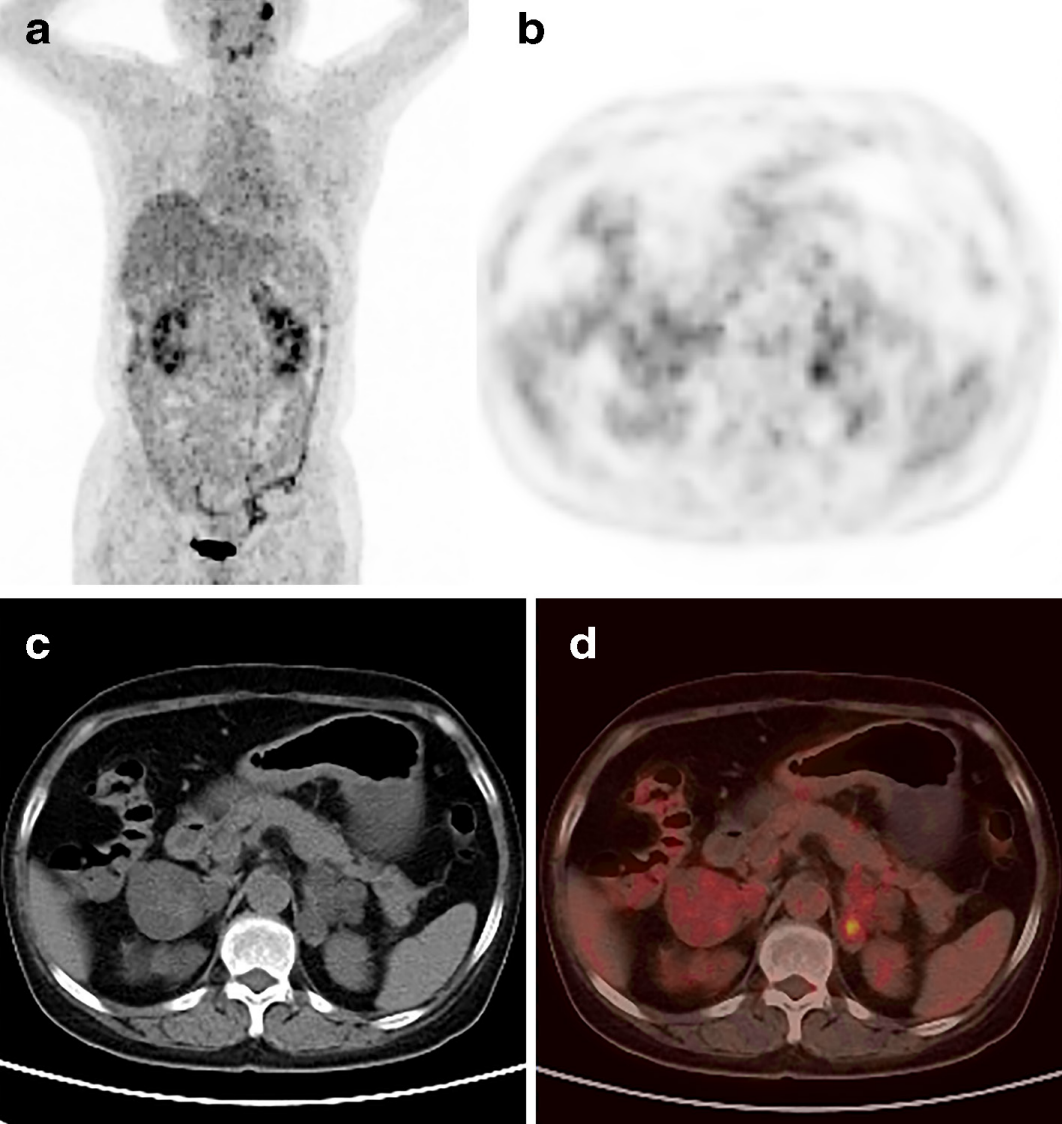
No obvious abnormality can be observed on the whole-body PET MIP image (A); Mild FDG uptake (SUVmax: 3.7) was observed in the right adrenal and left adrenal glands (SUVmax: 3.9) on axial PET (B); A cystic hypodensity with well-defined boundary and ginger-like appearance was observed on the adrenal gland with a CT value of 0 ~ 4 HU on the adrenal gland and 8 ~ 15 HU on the right adrenal gland on transversal CT (C); Bilateral adrenal lesions showed a slight increase in uptake on the fusion PET/CT images (D) PET: positron emission tomography, CT: computed tomography, MIP: maximum intensity projection, SUVmax: maximum standardised uptake value, FDG: fluorodeoxyglucose
